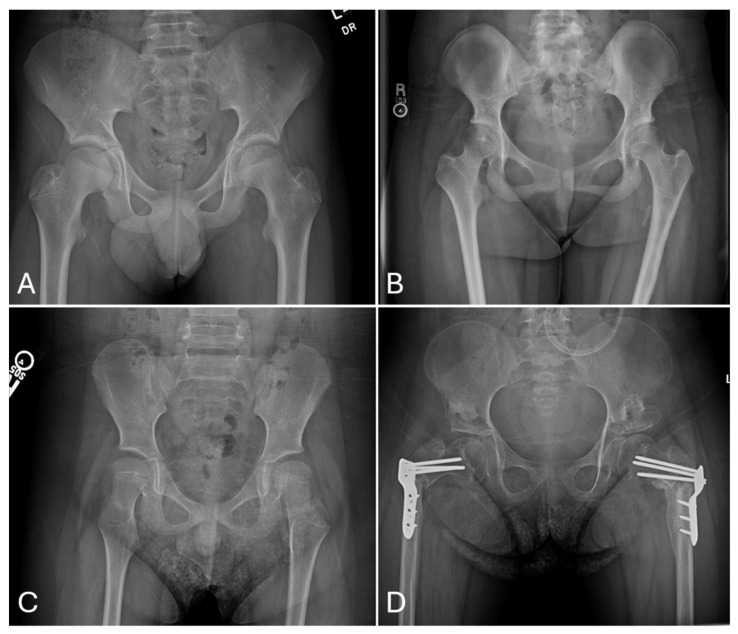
Representative anteroposterior pelvis radiographs used in the study. (A) 14-year-old male with GMFCS I CP. (B) 15-year-old female with GMFCS II CP. (C) 7-year-old male with GMFCS IV CP. (D) 9-year-old female with GMFCS V CP, 3 months after hip reconstruction.
